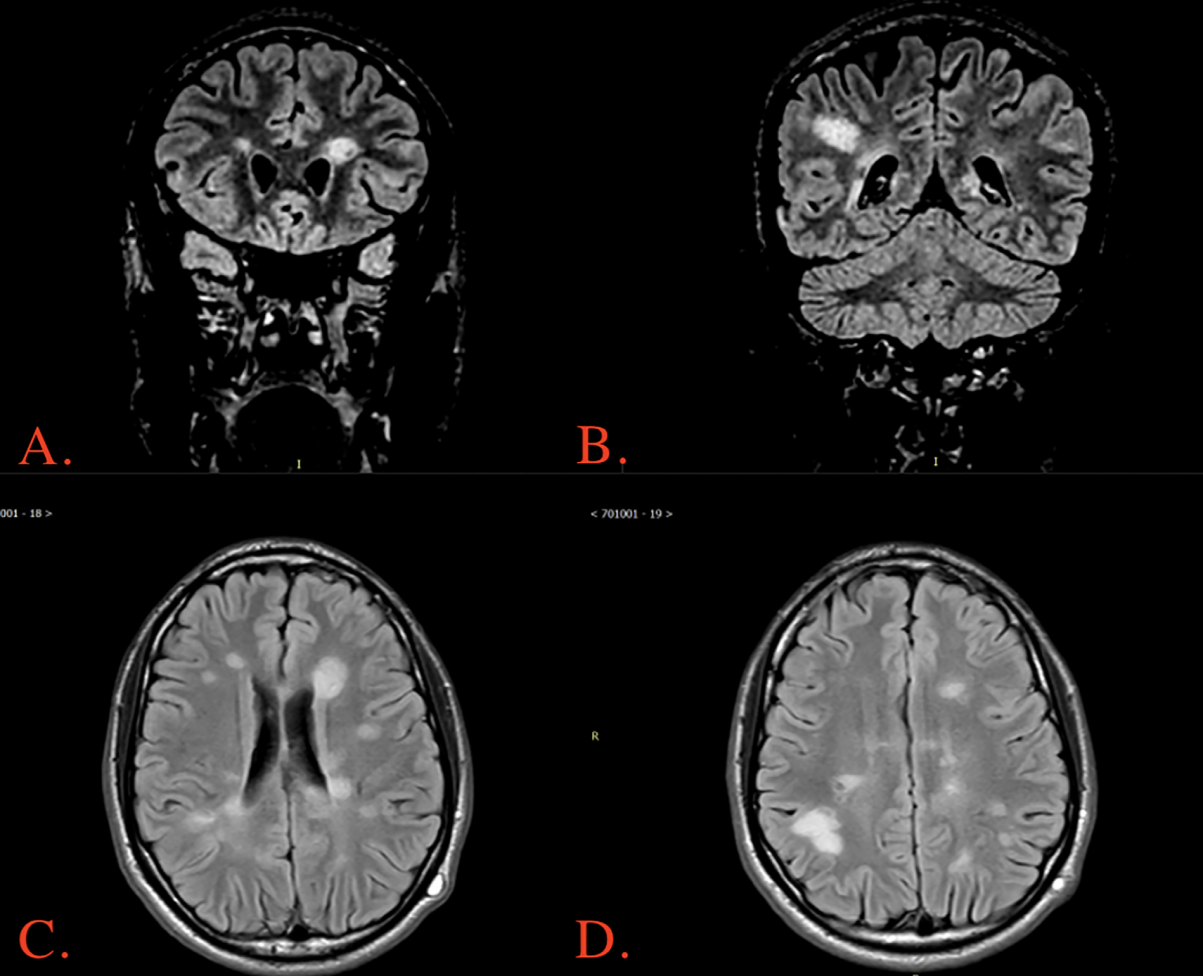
(A and B) Coronal FLAIR showing T2 signal intensity affecting both the supratentorial and infratentorial regions of the brain (C and D) Axial FLAIR showing T2 signal intensity affecting both the supratentorial and infratentorial regions of the brain.
